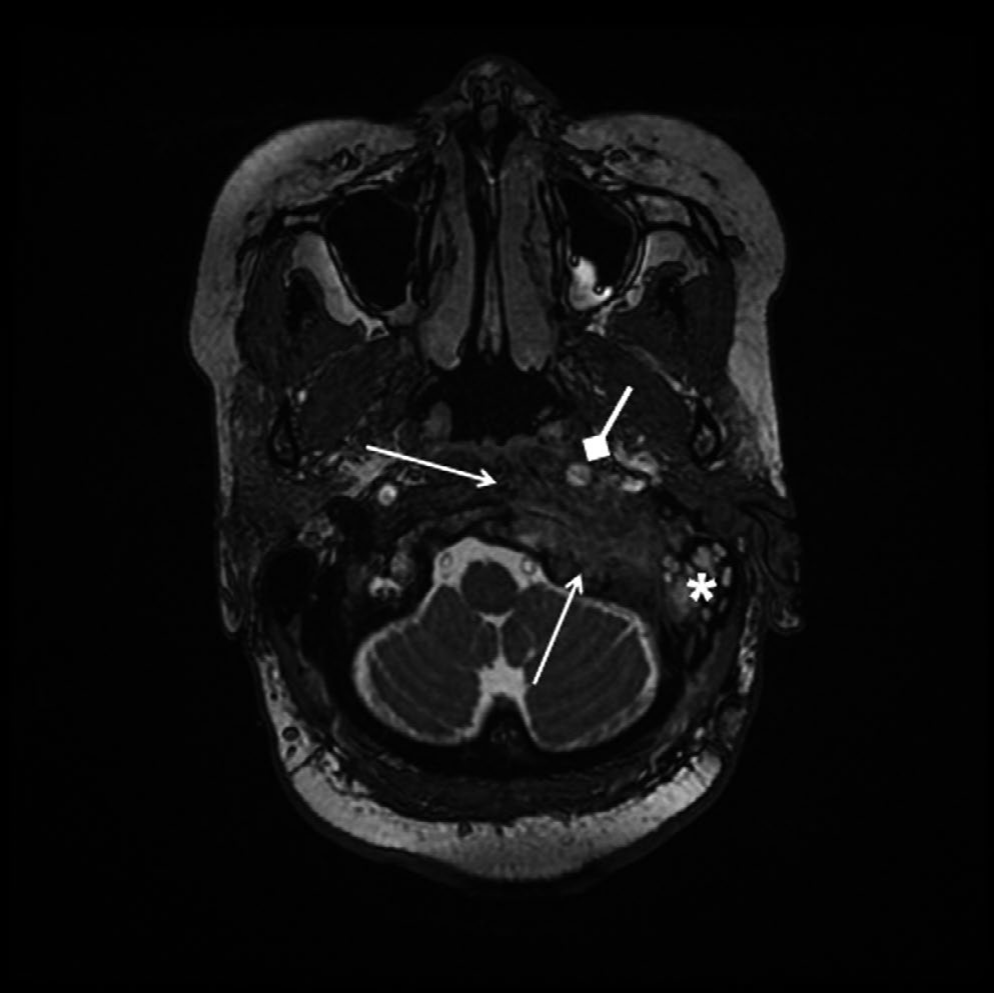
T2‐weighted thin‐slice fast imaging employing steady‐state acquisition (FIESTA) magnetic resonance imaging (MRI) sequence shows left clival and petrous bone T2 hypointense mass (open arrows) displacing the left internal carotid artery anteromedially (diamond arrow), with left mastoid effusion (asterisk)
